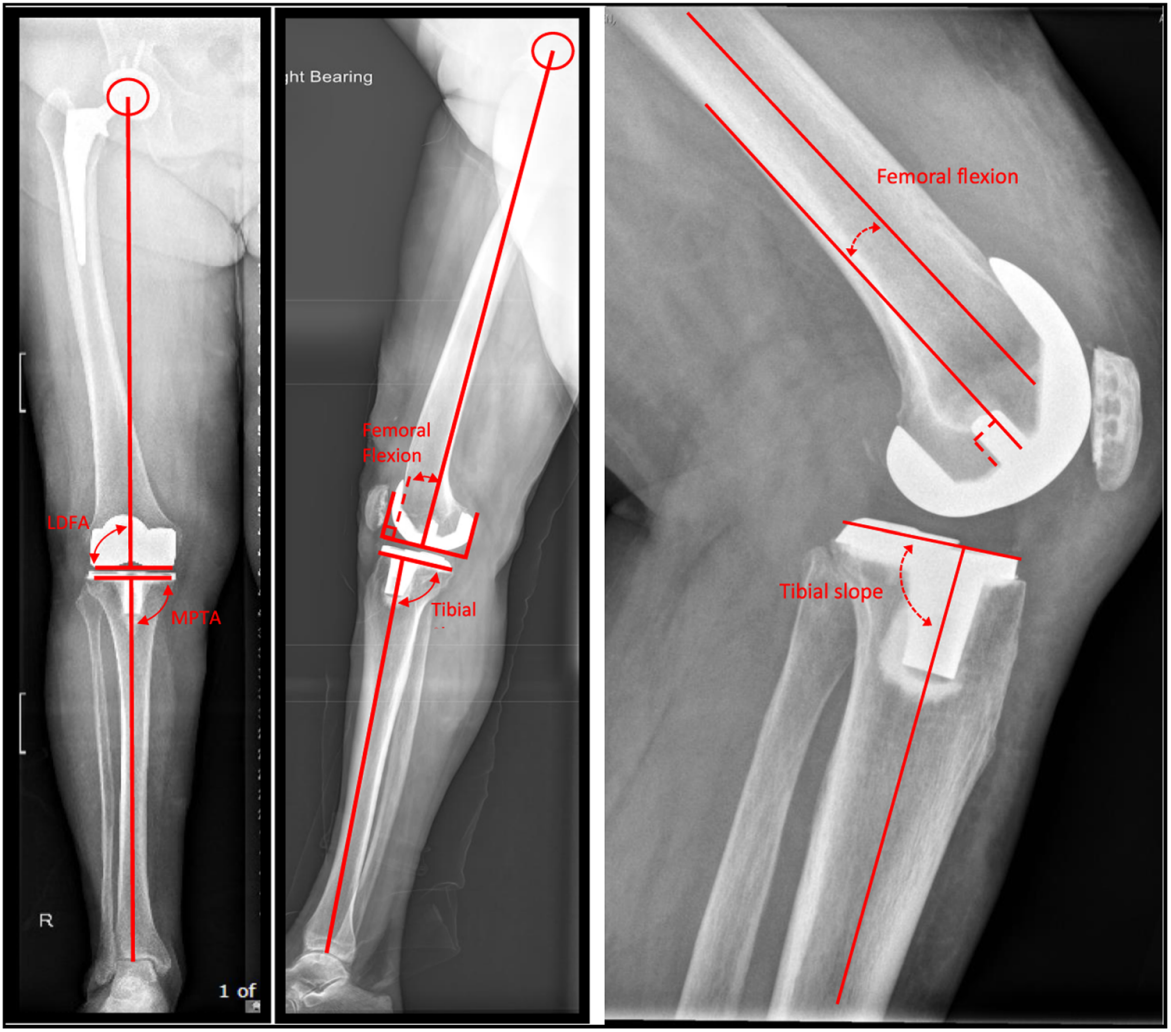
Measurement of postoperative long‐leg coronal and sagittal and short‐leg sagittal radiographs as previously described [18]. Coronal plane alignment (lateral distal femoral angle [LDFA] and mechanical medial proximal tibial angle [MPTA]) was evaluated on long‐leg anteroposterior radiographs. Sagittal plane alignment (femoral flexion and tibial slope) was evaluated on short‐leg and long‐leg lateral radiographs.
